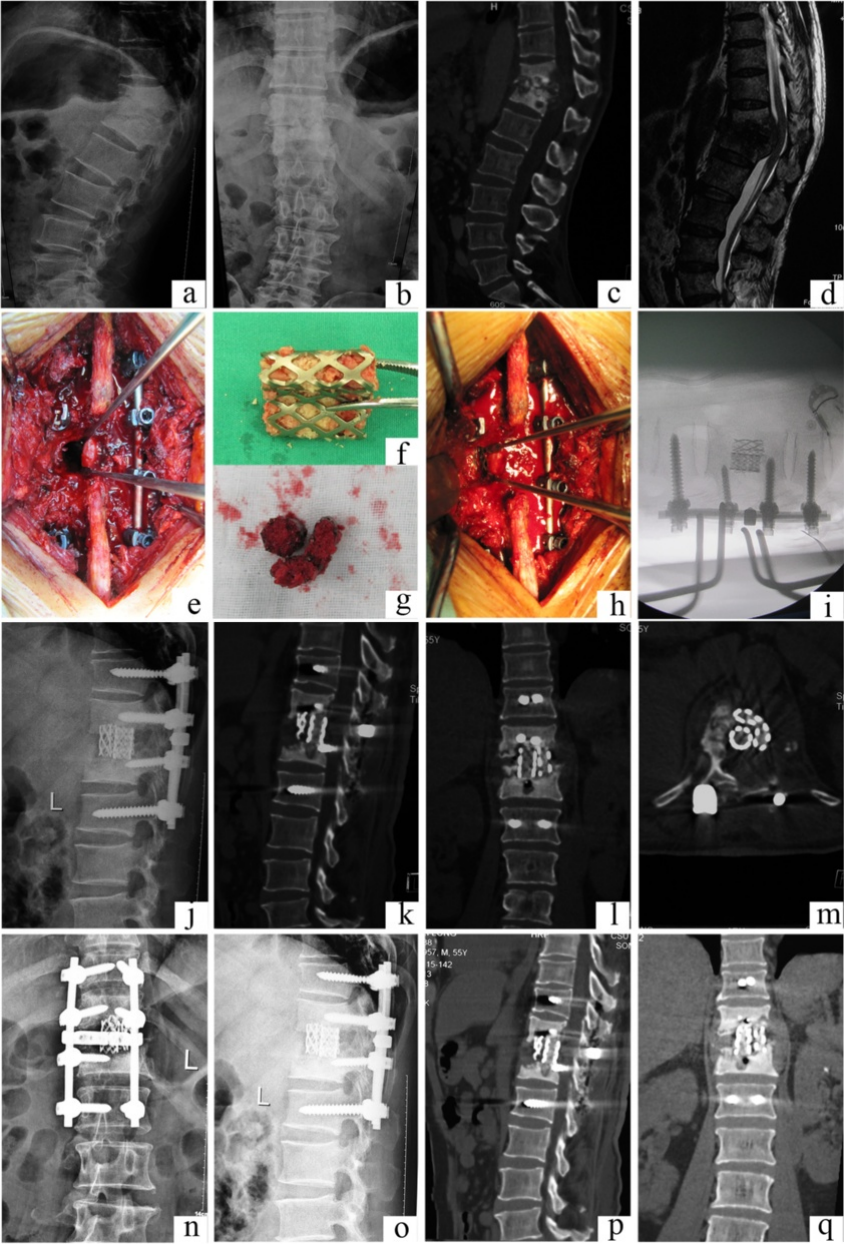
Case number 2. Preoperative image evaluation. a, b Radiograph showing T11–12 involvement. c Computed tomography (CT) and d magnetic resonance imagery (MRI). The sagittal T2-weighted MRI showed a T11 vertebral mass with collapse and kyphosis, compressing the spinal cord. e–i Operation technique and specially formed titanium mesh cages. j Postoperative radiograph. k–m Postoperative CT showing the kyphosis is correct. n–q The latest follow-up at 48 months postoperatively showed that the internal fixation was in good shape, interbody fusion had been obtained, and there were no signs of tuberculosis recurrence
